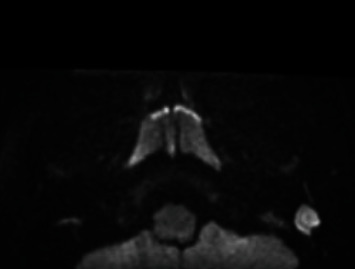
Magnetic resonance DWI sequences showing recurrence of cholesteatoma in the left ear. DWI, diffusion weighted imaging.
